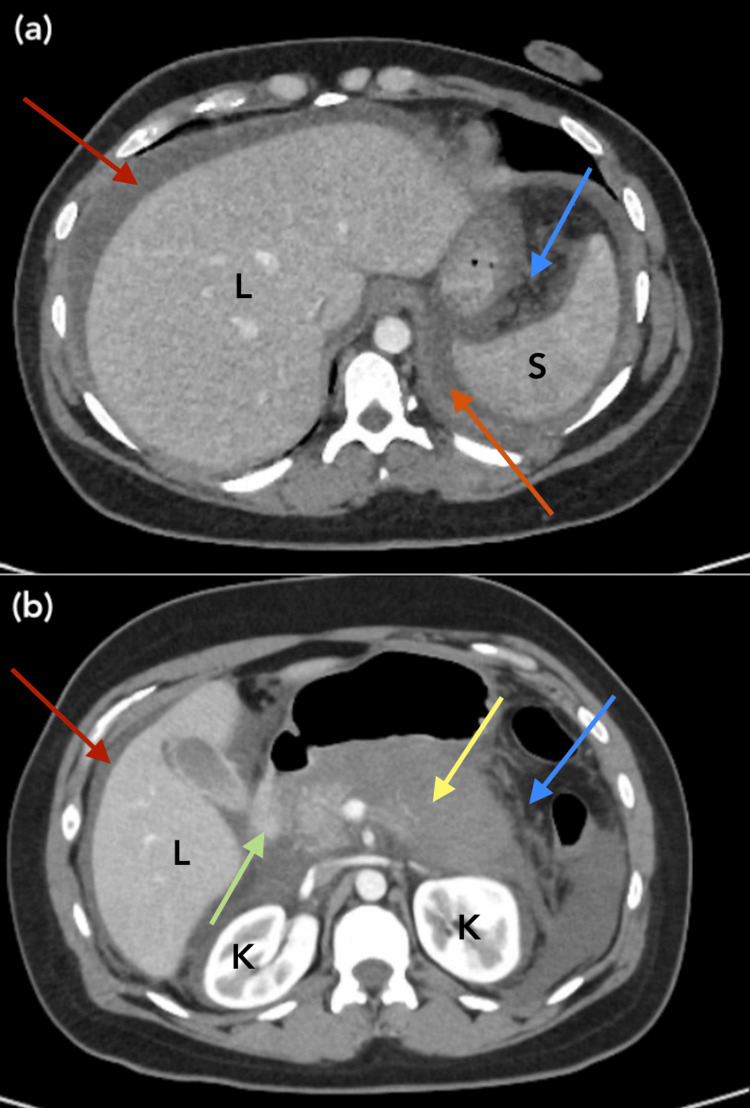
Representative images (a, b) of CTAP w/IV contrast from CUH day two. Note the poorly defined nature of the pancreatic body (yellow arrow) due to the extensive, damaging necrotizing process L: Liver; S: Spleen; K: Kidney Red arrow: Perihepatic ascites Orange arrow: Pronounced fluid collection tracking near the spleen, consistent with peripancreatic edema Yellow arrow: Poorly-defined, markedly hypoenhancing pancreatic body and tail, consistent with acute necrosis Green arrow: A more normal-appearing/properly enhancing area of pancreatic head tissue, in stark contrast to the hypoenhancement of the remainder of the pancreas Blue arrow: Peripancreatic edema and fat stranding secondary to necrosis Abbreviations: CTAP: Computed tomography of abdomen/pelvis; IV: Intravenous; CUH: Cooper University Hospital
